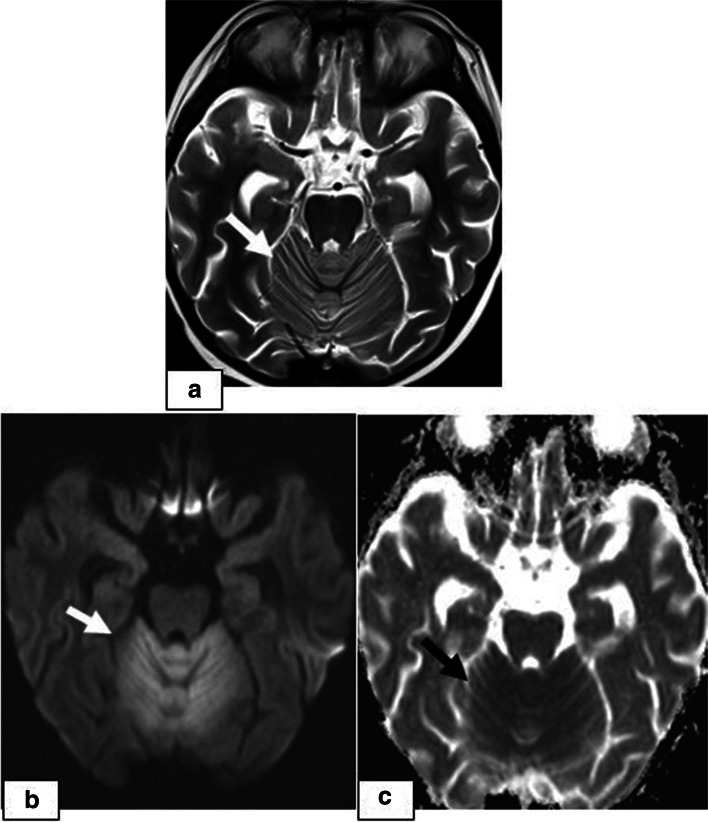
A 7-year-old girl presenting with seizures and headache 37 days after undergoing allogeneic HSCT for haemophagocytic lymphohistiocytosis. a Axial T2W image showing abnormal cerebellar hyperintensity (arrows). b DWI and c) ADC images showing diffusion restriction (arrows). She died 3 weeks after PRES onset due to acute pulmonary GVHD
